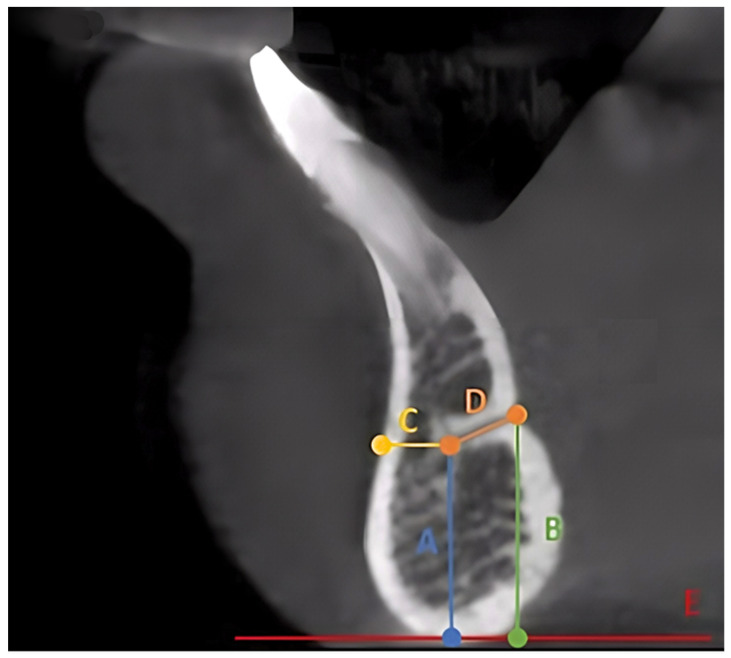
CBCT sagittal cut photograph showing study variable measurements: (A) Inferior border to the buccal terminal; (B) inferior border to the lingual terminal; (C) buccal cortex to the buccal terminal; (D) canal length; (E) lower border reference line.
